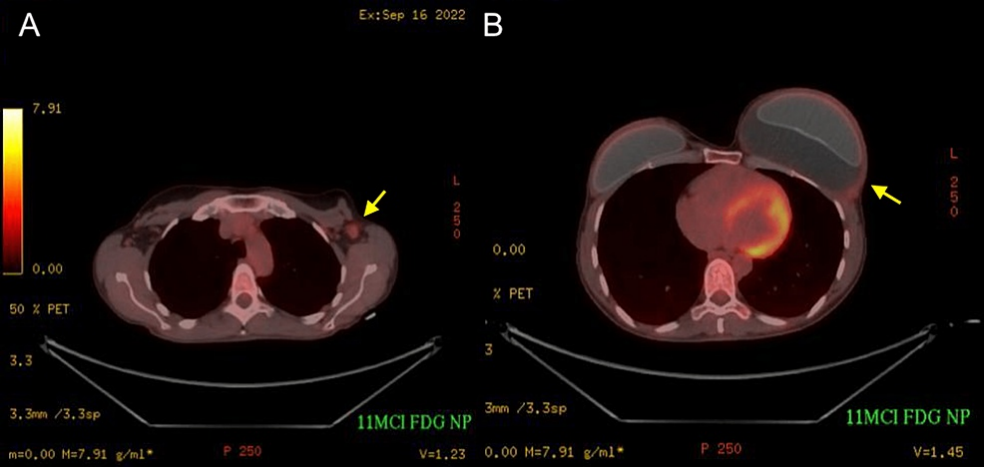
(A) Axial fluorodeoxyglucose positron emission tomography-computed tomography image demonstrating FDG-avid enlarged left axillary lymph node. (B) Axial FDG positron emission tomography-computed tomography image demonstrating only minimal fluorodeoxyglucose avidity of the peri-implant collection capsule wall.
